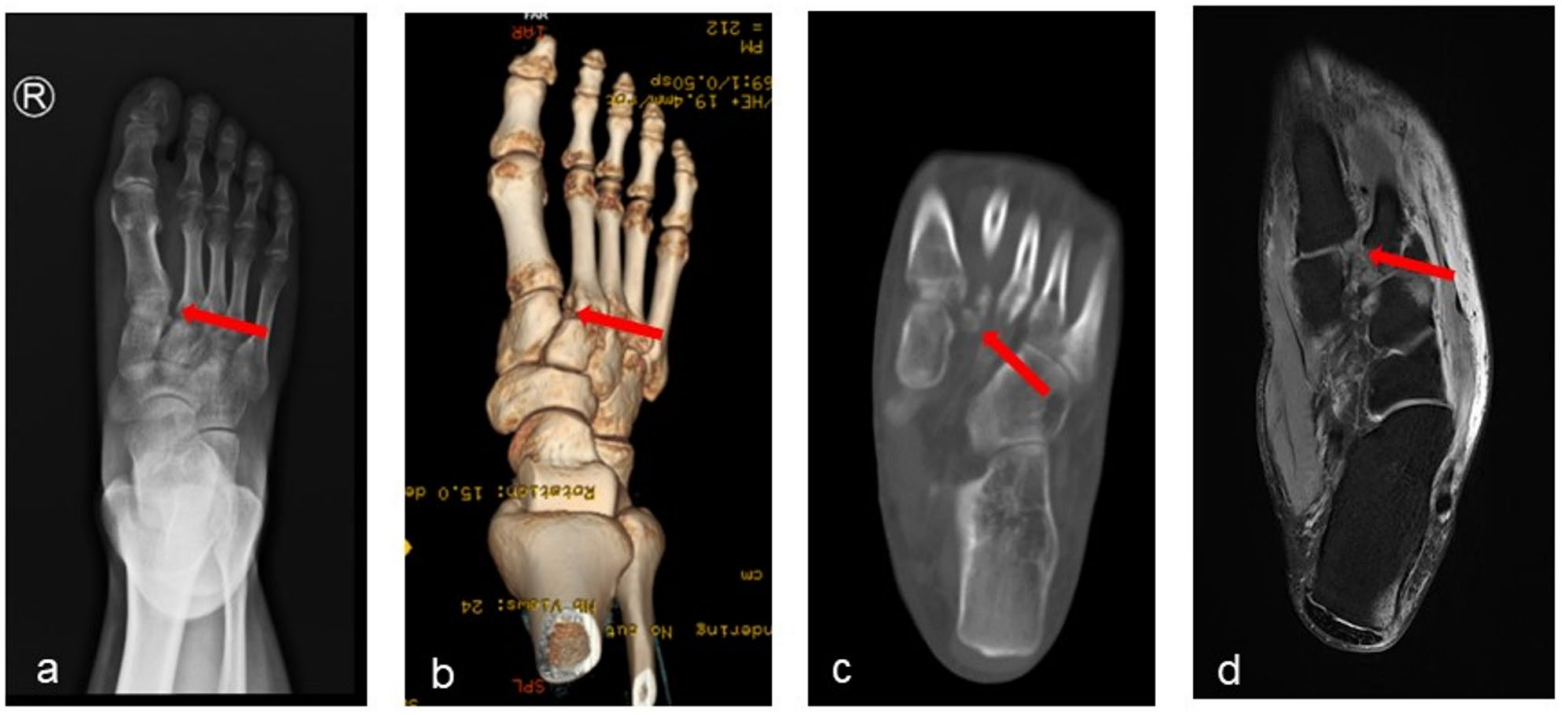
Imaging findings of Lisfranc injury : (a) The weight-bearing anteroposterior view of the foot shows a significantly widened gap between the second metatarsal and the medial cuneiform. b 、c CT scans reveal a significantly widened gap between the second metatarsal and the medial cuneiform, accompanied by an avulsion fracture at the base of the second metatarsal. d MRI shows a complete rupture of the Lisfranc ligament
